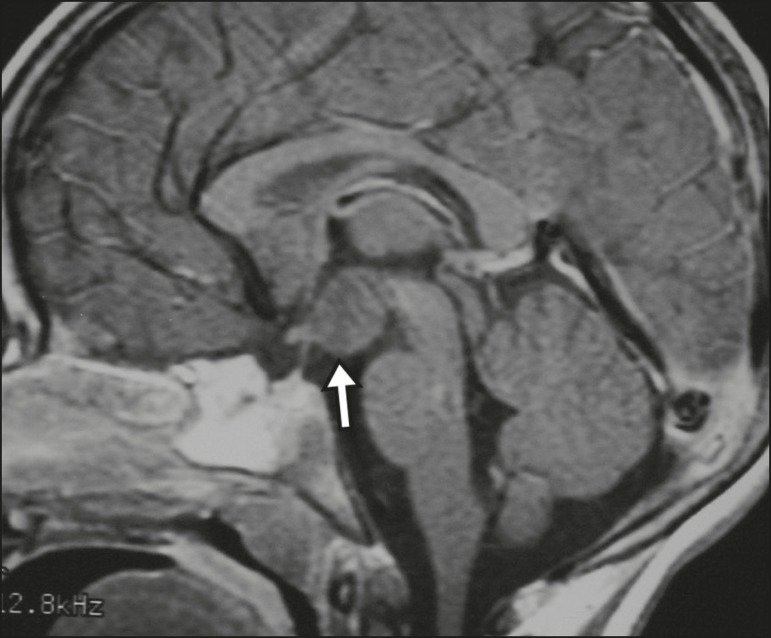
Sagittal T1-weighted MRI sequence showing an intrahypothalamic hamartoma
